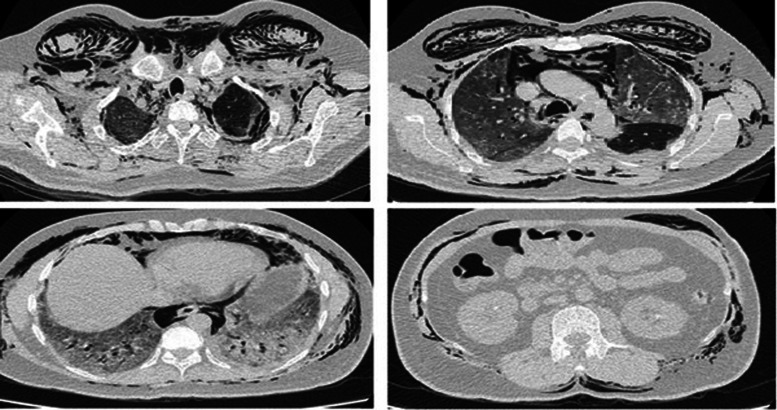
CT chest and abdomen showing extensive SE and pneumoperitoneum.
